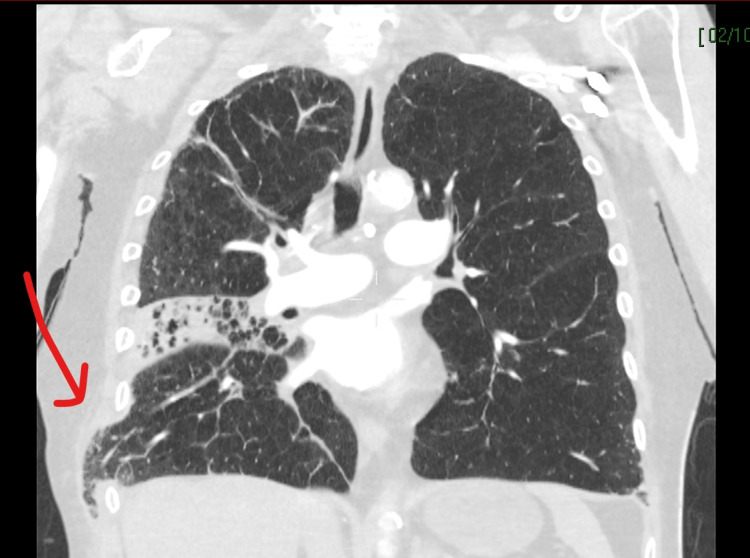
CT chest (coronal view) demonstrating right lateral lung herniation below the eighth rib and middle lobar consolidation. Red arrow pointing to lung herniation.
